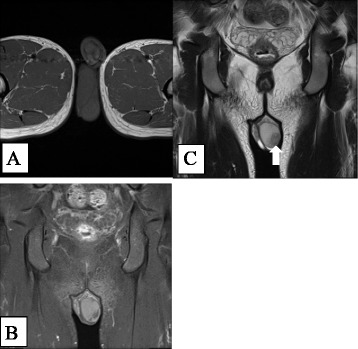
a Axial T1-weighted magnetic resonance image shows that the lesion has an isointense signal. b After administration of gadolinium contrast, material internal enhancement is not observed. c Coronal T2-weighted magnetic resonance image shows that the lesion has low signal intensity (*arrow*)
